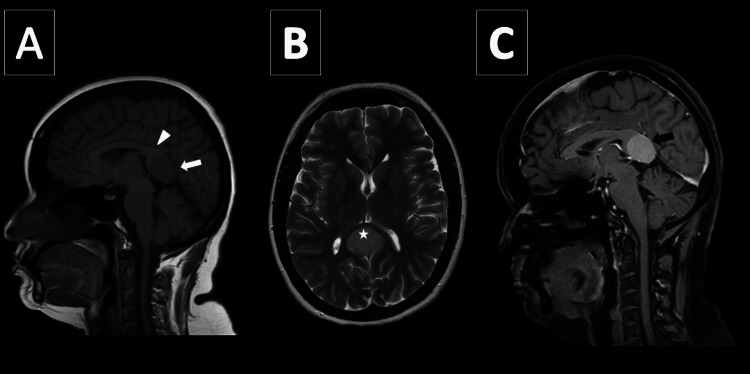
Case 2, a 30-year-old man. T2-weighted and pre- and postcontrast T1-weighted sequences. (A) The sagittal precontrast T1-weighted image shows a well-defined, extra-axial, supratentorial mass centered in the pineal region (arrow) that is isointense to gray matter and compresses the splenium of the corpus callosum (arrowhead). (B) The lesion is mildly hyperintense on T2-weighted images. (C) The sagittal postcontrast fat-suppressed T1-weighted image shows the mass homogeneously enhances.
